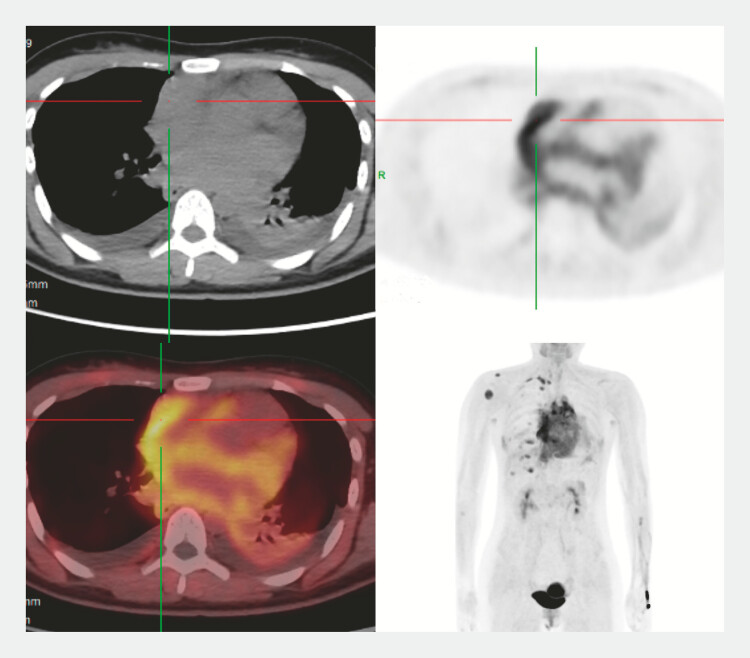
PET/CT showed diffuse metabolic hyperactivity in the heart and local mediastinum, which was considered suspicious for malignancy. PET/CT, positron emission tomography/computed tomography.
